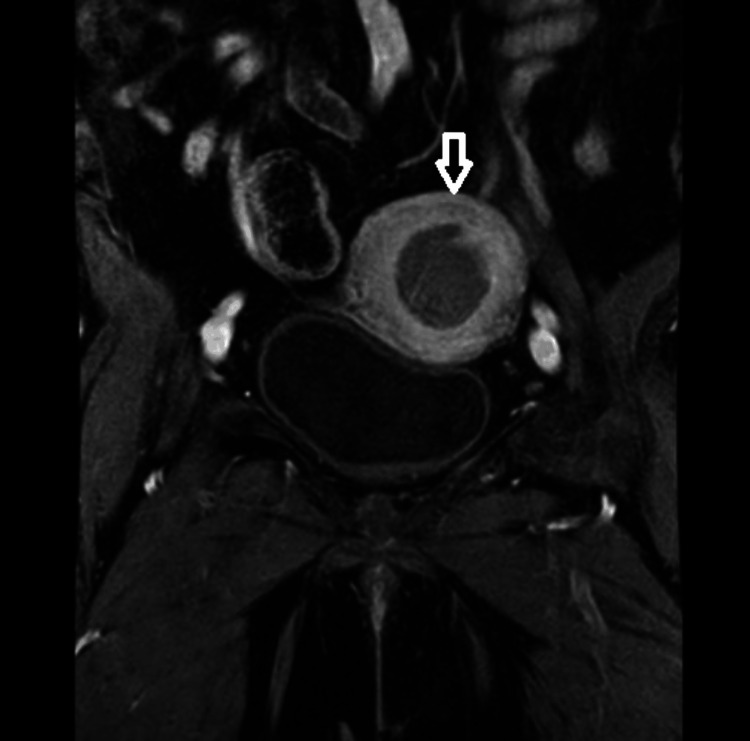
Coronal T1-weighted contrast-enhanced MRI image showing a lesion in the fundic region on the left side MRI: magnetic resonance imaging
